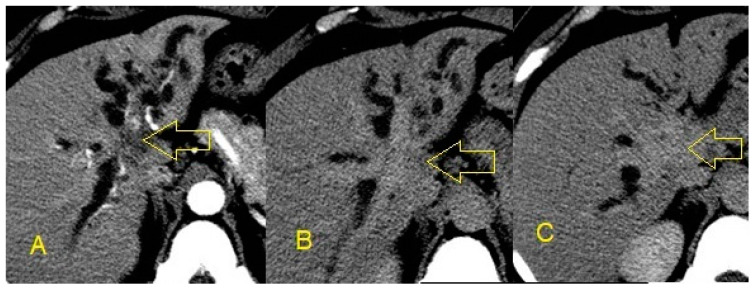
A: Axial arterial phase CT shows dilated intrahepatic ducts and a hypodense mass (arrow) at the hilum; B,C: venous phase images show enhancement of the mass. This signifies excessive fibrous stroma in the tumor and is consistent with cholangiocarcinoma.
